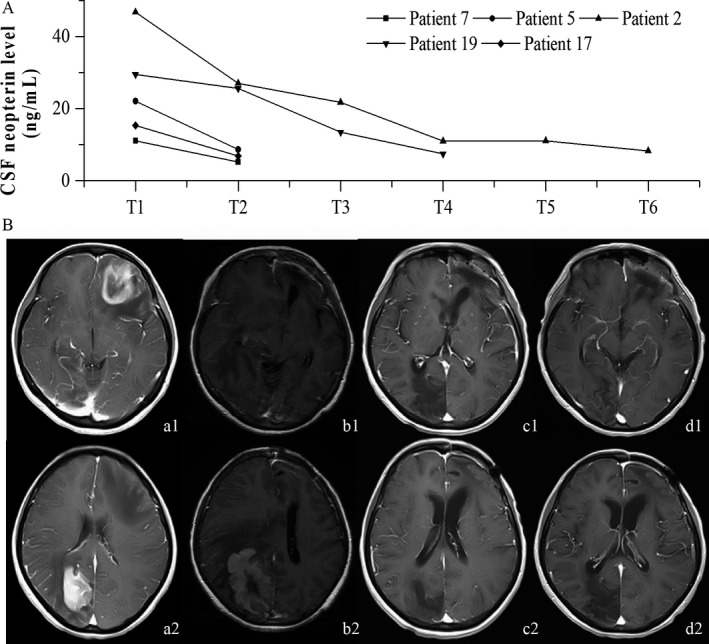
Monitoring tumor dynamics with CSF Npt concentration. A, Changes in the CSF Npt concentration before (T1) and immediately after (T2) surgery and at 1 (T3), 3 (T4), 6 (T5), and 9 (T6) months after surgery in 5 patients (Cases 2, 5, 7, 17, and 19). B, MR images and CSF Npt concentration in a 49‐y‐old woman with PCNSL
